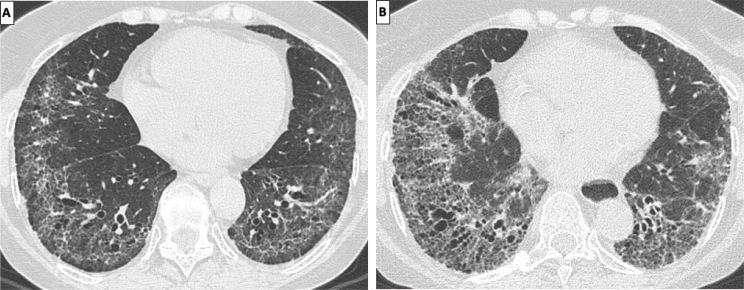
Axial HRCT images of a SSc-NSIP (baseline evaluation in A) showing evidence of fibrosis progression at 3-year follow-up (B), mostly demonstrated by an increased extent of traction bronchiectasis and reticular opacities. HRCT, high resolution computed tomography; SSc-fibrotic NSIP, systemic sclerosis-fibrotic nonspecific interstitial pneumonia
